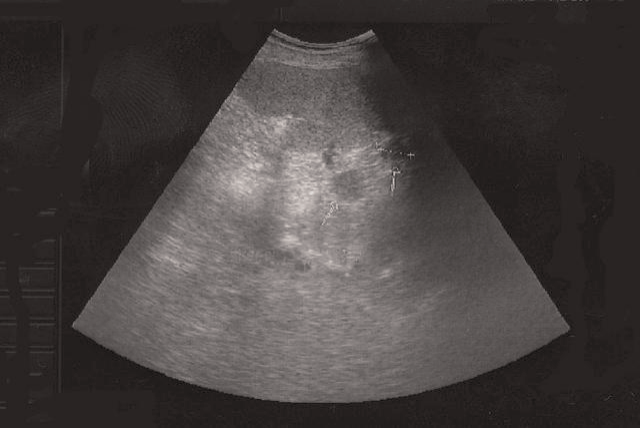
Ultrasound shows two nodular lesions consistent with polysplenia (arrows).
